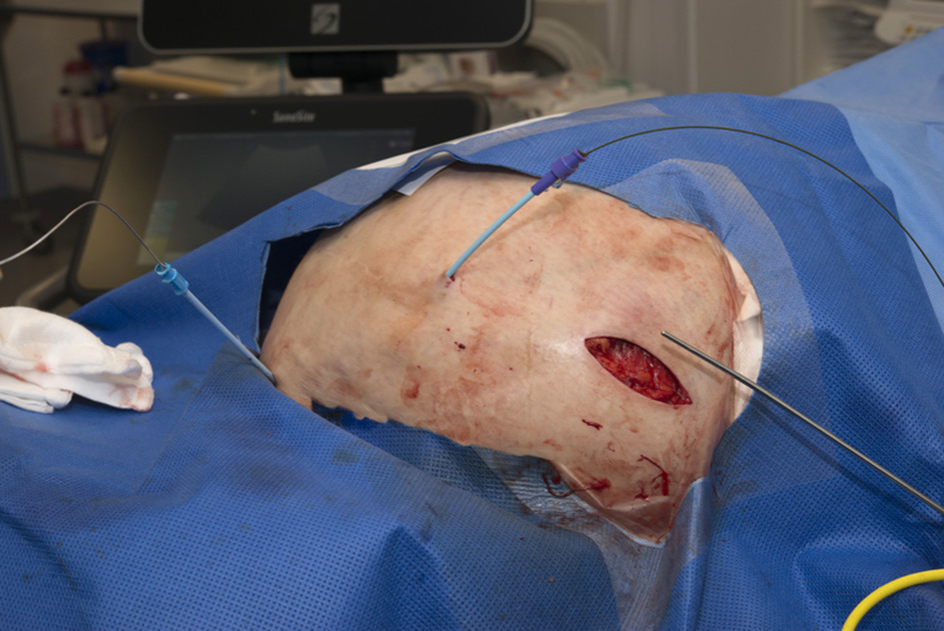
Dilators and guide wires in the urinary bladder and peritoneum following needle access under ultrasound. The metallic dilator overlies the subcutaneous pocket for the control unit.
